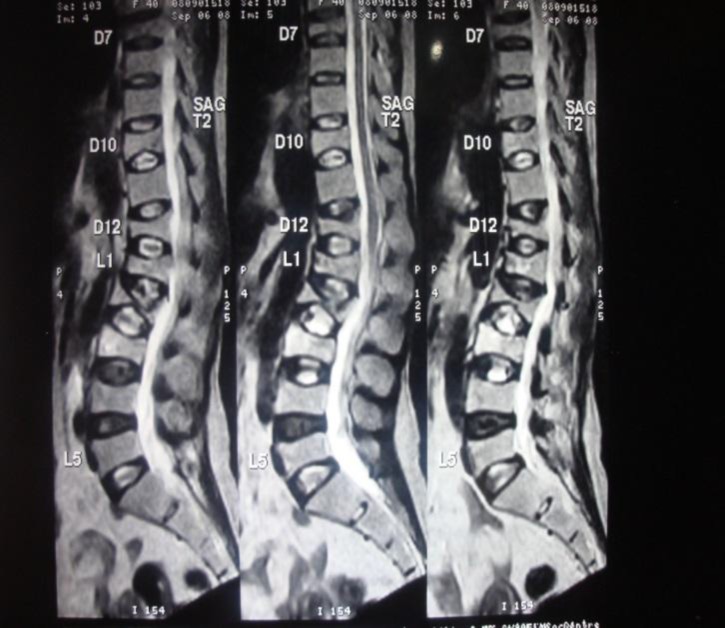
MRI- decreased height of vertebral body suggestive of collapse of T7, 10, 12 and L1-3.
